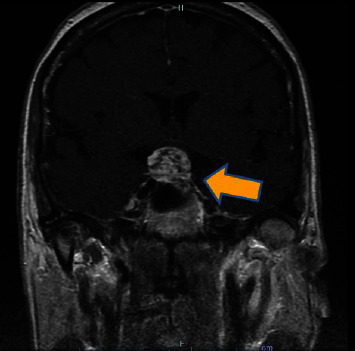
The T1-weighted coronal image shows an enhancing mass that invades the cavernous sinus, indicating a mass measuring 1.9 cm × 1.9 cm.
